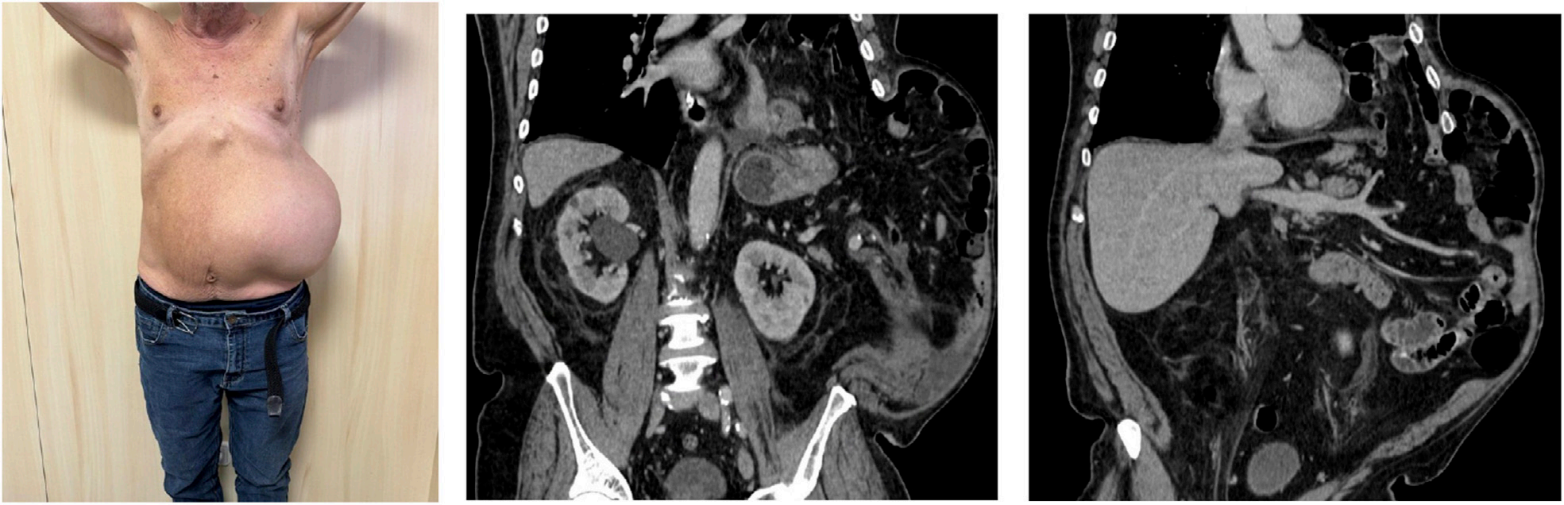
Patient and preoperative CT scan.
